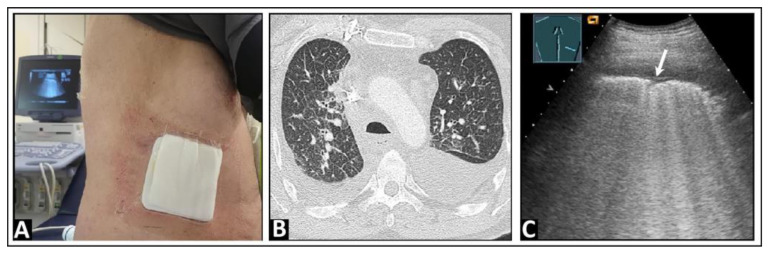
(A) A patient with an indwelling pleural catheter in place for 6 weeks due to a malignant effusion from a bronchial carcinoma and with exertion and speech dyspnea. (B) On chest computed tomography, multiple nodular consolidations are visualized, consistent with metastases, as well as septal thickening (right > left) indicative of lymphangitic carcinomatosis. (C) On lung ultrasound, the “white lung” pattern is observed, with a rough lung surface (arrow) and a delicate anterior pleural effusion.
